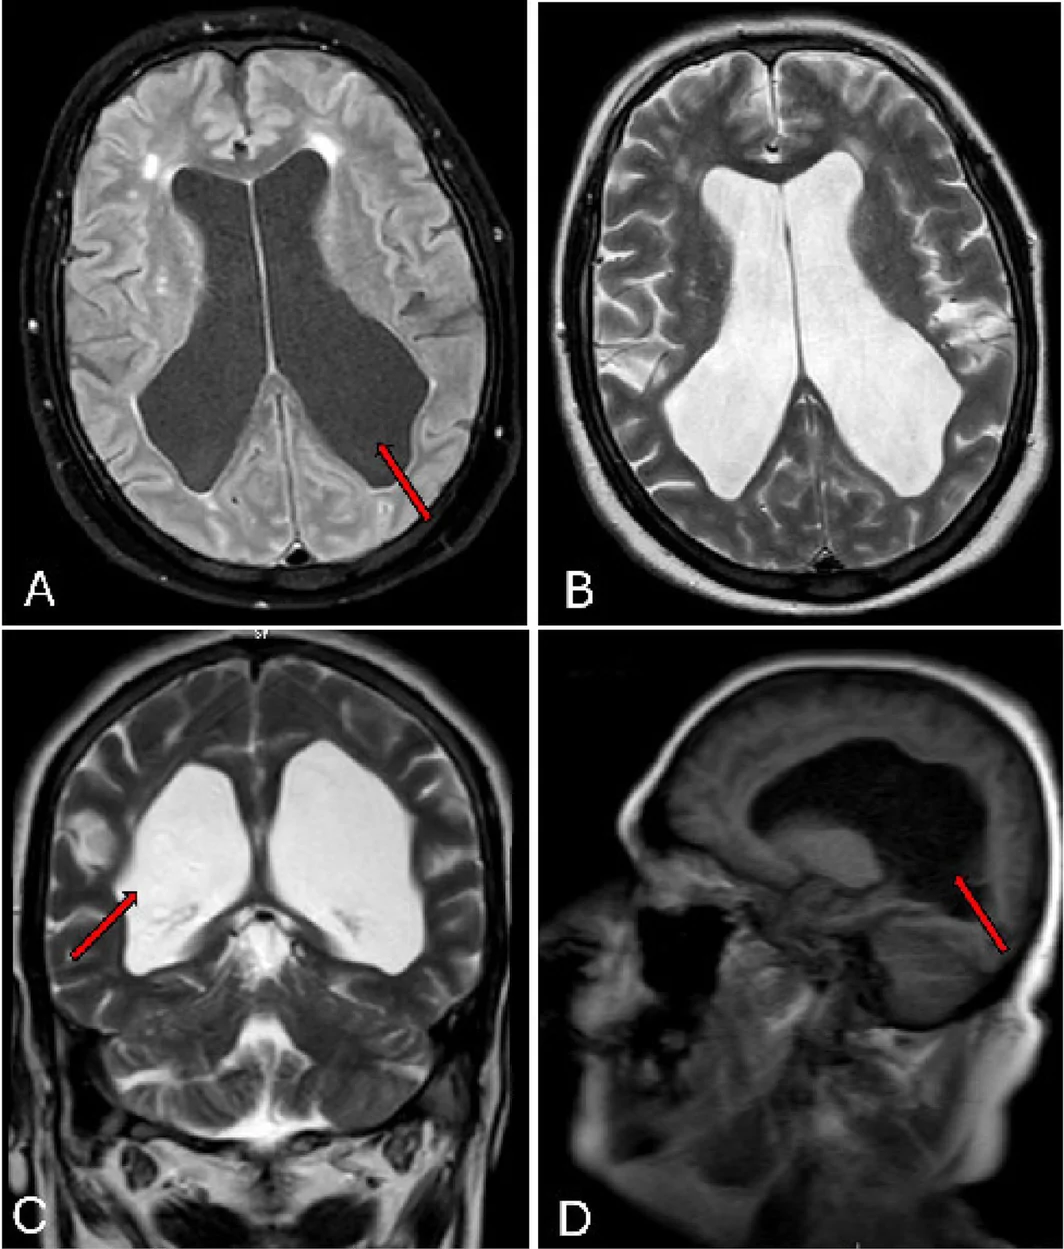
T1(A) and T2(B)‐ weighted brain MRI show moderate to severe two‐ventricle hydrocephalus (both lateral ventricles) in transverse (A and B), coronal (C), and horizontal (D) views (red arrows).
